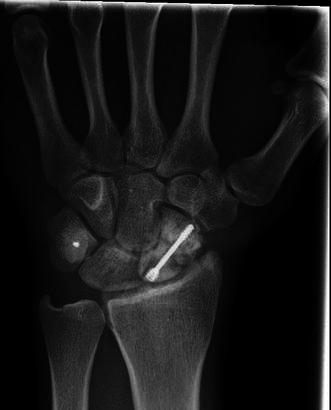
Wrist PA X-ray at 12 months suggesting non-union of scaphoid fracture
